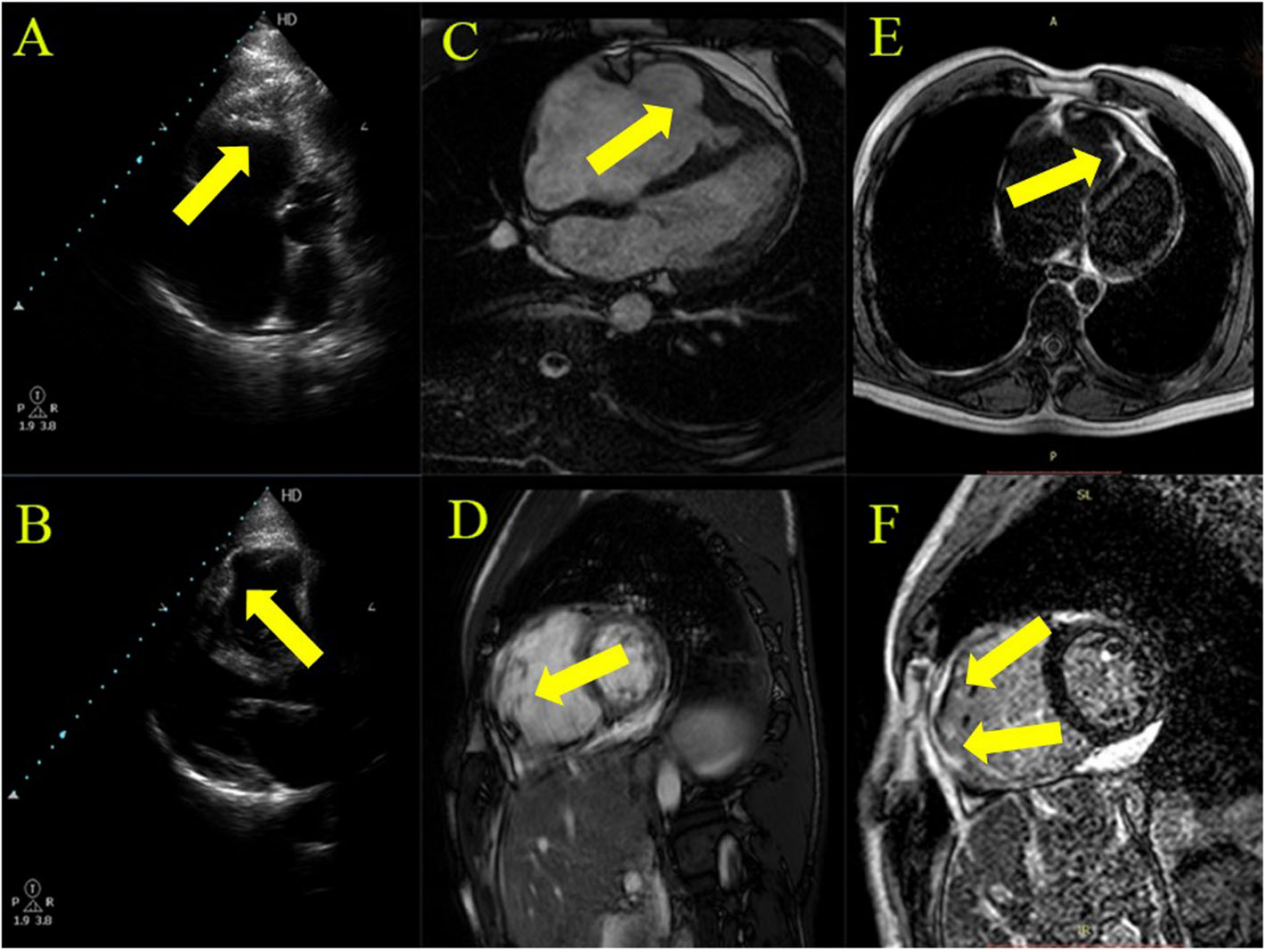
TTE and CMR of EMF. a 2D TTE, RV apical four-chamber modified view showed obliteration of the RV apex (marked with arrows). b 2D TTE, parasternal long axis view showing dilated RV dimensions (marked with arrows). c, d Cine CMR apical four-chamber and short axis views showing dilated RV dimensions (marked with arrows). e, f LGE CMR imaging showing subendocardial LGE enhancement at the RV apex and RV free wall (marked with arrows)
